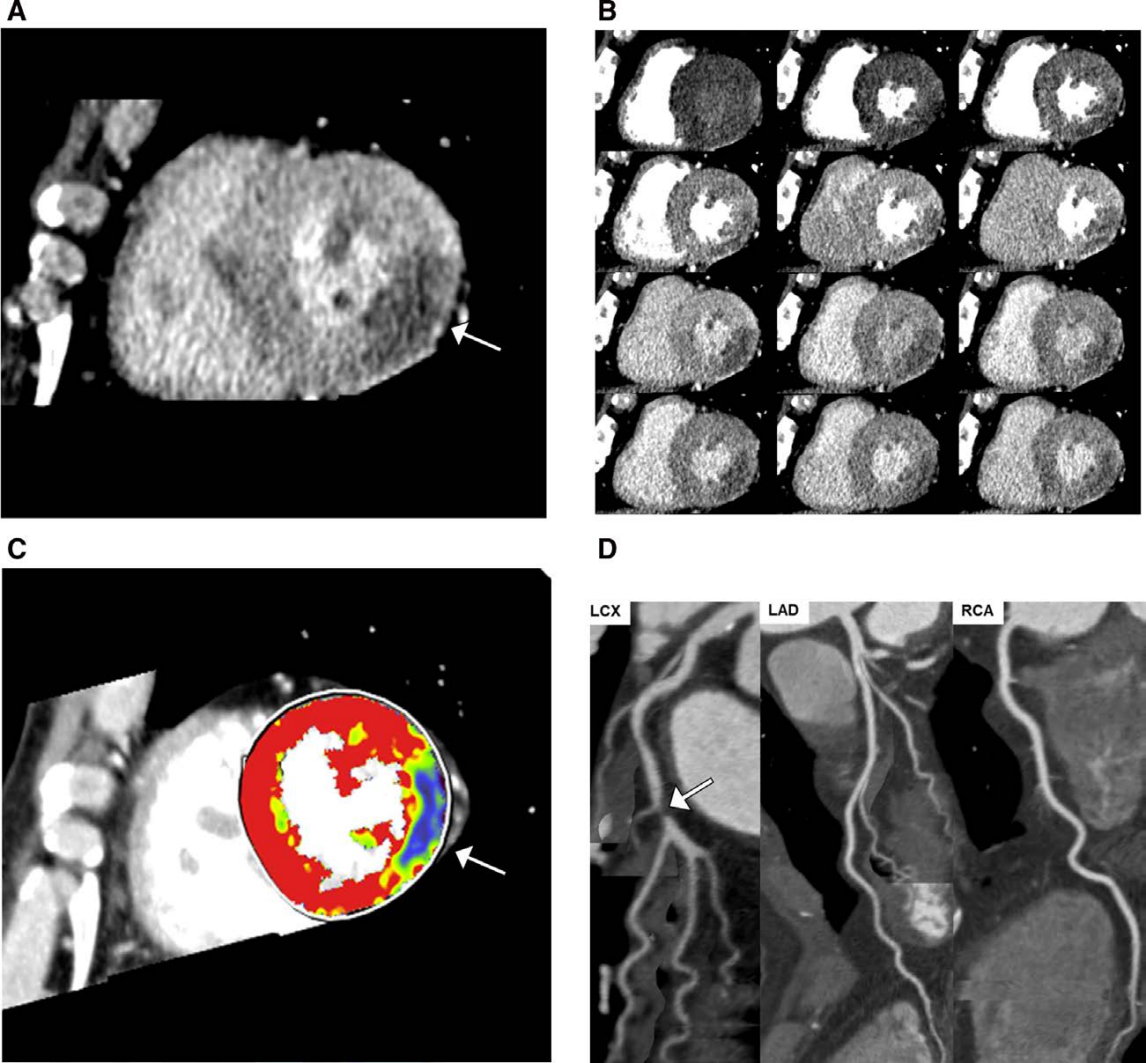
Stress CT myocardial perfusion images in a 53-year-old male patient with distal left circumflex artery stenosis. Virtual static CTP image (A) and dynamic CTP images (B and C) show myocardial perfusion defects in the anterior lateral wall of the middle left ventricle. Virtual static CTP image (A) demonstrates a perfusion defect (arrow) measuring 58.2 HU. Color map (C) shows the reduced myocardial blood flow (31.1 mL/100 mL/min, arrow) in the left circumflex artery territory. Coronary CT angiography (D) demonstrates severe coronary artery stenosis (arrow) in the left circumflex artery. FFR was not measured in the distal left circumflex artery because the degree of stenosis was >90%. CTP = computed tomography perfusion, FFR = fractional flow reserve, HU = hounsfield unit.
